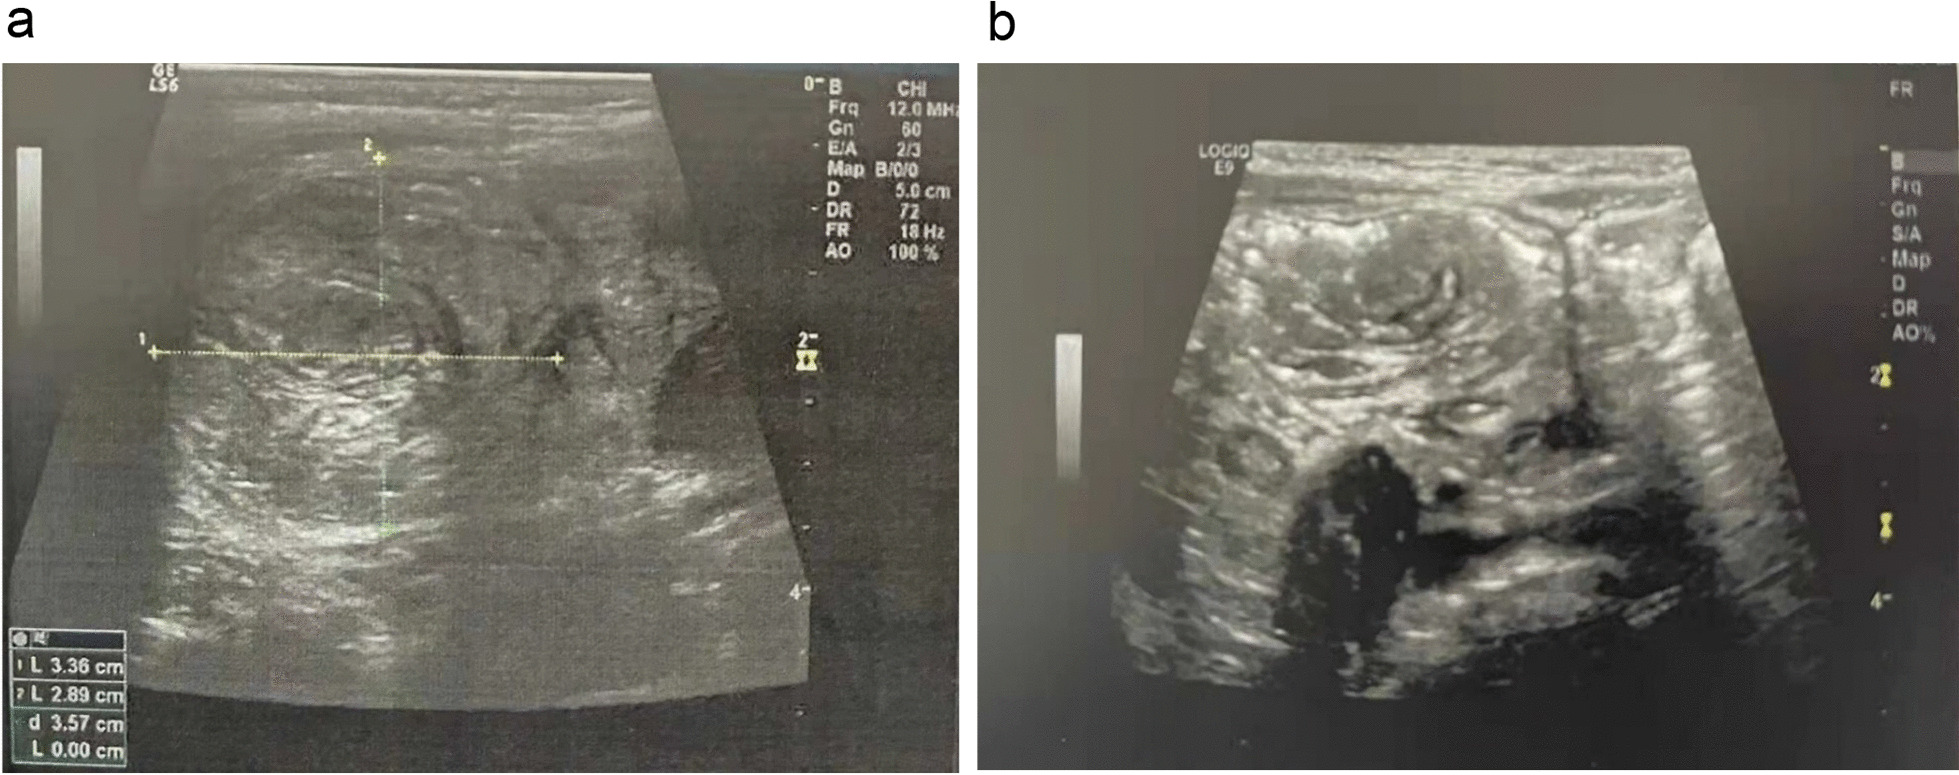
Ultrasound examination before and after enema reduction. A hypoechoic ring was seen (panel a) by ultrasound, a feature of intussusception, before enema reduction, which disappeared after reduction (panel b)
